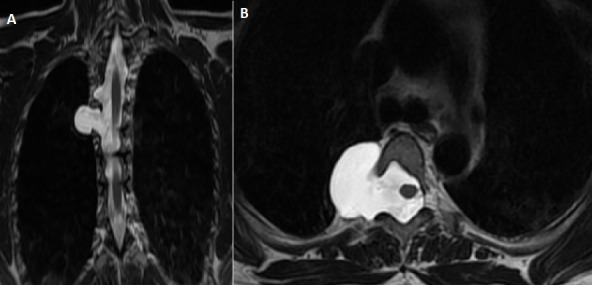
coronal (A) and axial (B) T2-weighted spin-echo magnetic resonance image of the dorsal spine showing anterior-meningocele D3-D4
